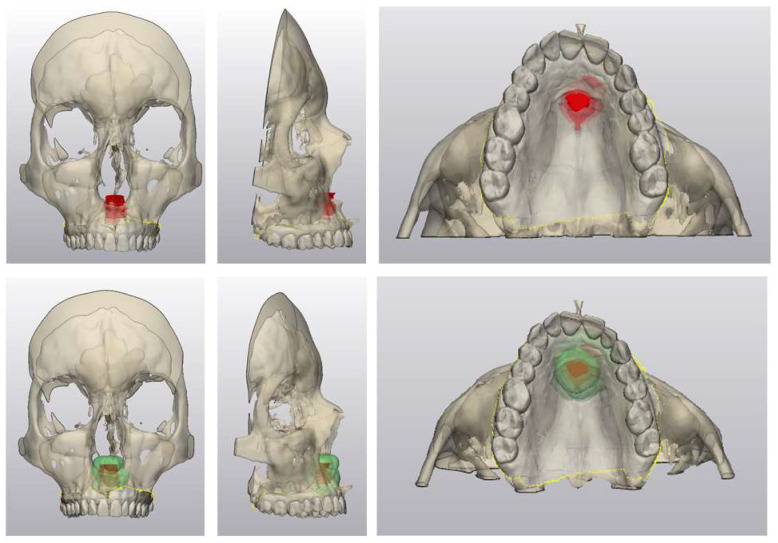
Virtual surgical planning based on CT–MRI fusion images, showing 3D segmentation of the tumor (red) and planned resection margins (green). Fusion of CT and MRI allows precise delineation of the palatal chondrosarcoma (red). The green volume represents the planned osteotomy margin that will be resected en bloc together with the tumor to achieve oncologic clearance. Patient-specific cutting guides were designed based on this model.
